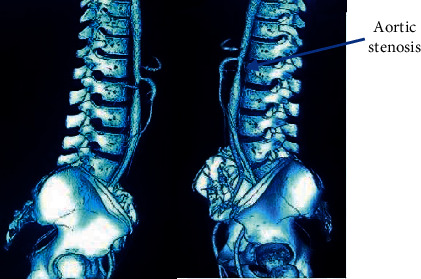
CTA showing aortic stenosis at L2 level.
